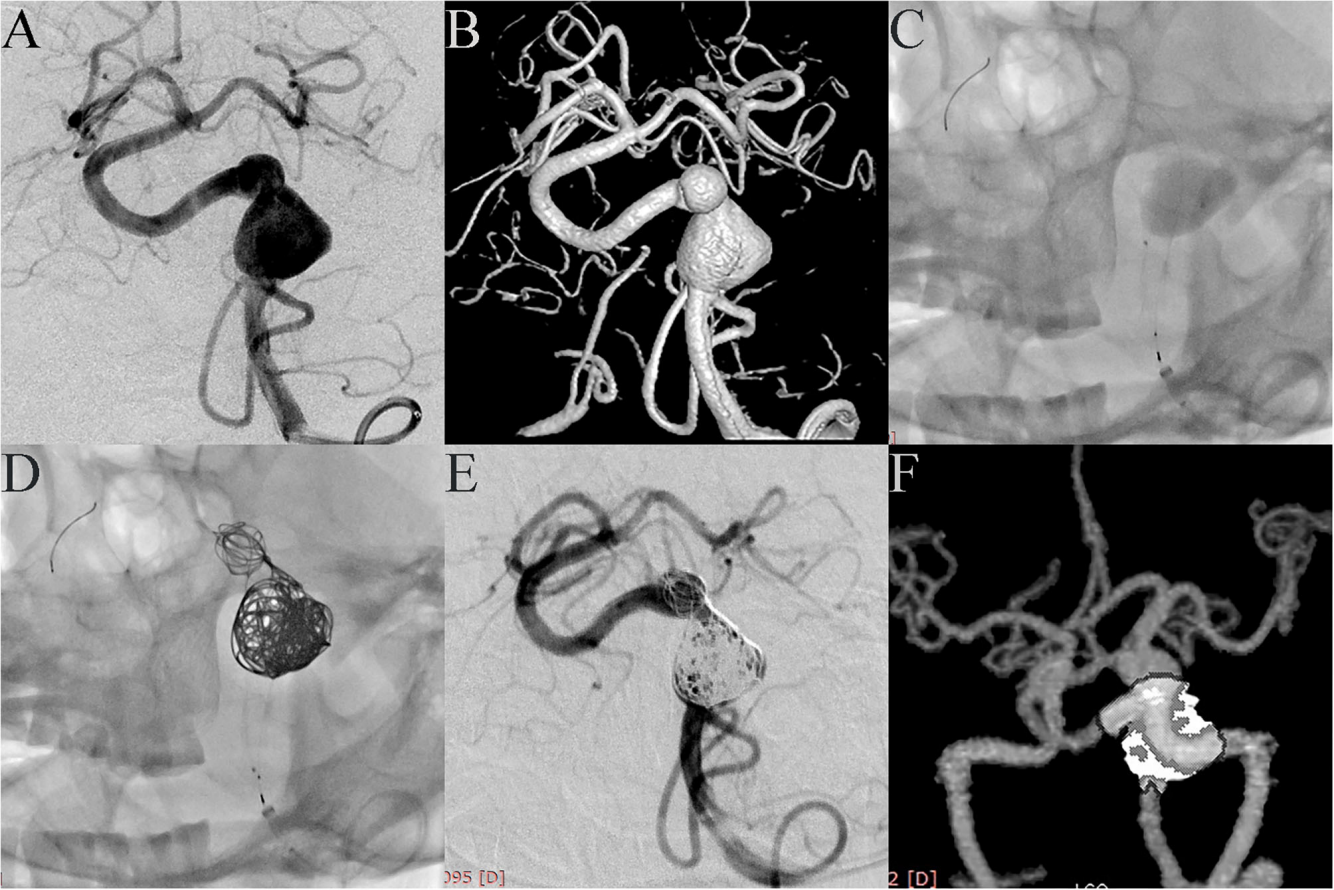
Case 4: Two adjacent fusiform aneurysms were found in the left vertebral artery. (A, anteroposterior projection by DSA; B, three-dimensional reconstruction). One PED was deployed along the left vertebral artery (C), and both aneurysmal necks were completely covered. Two aneurysm domes were then packed with coils (D). Post-embolization angiograms showed good vascular reconstruction. The large aneurysm was almost completely embolized and the small aneurysm showed significant contrast stasis (E). Follow-up at 10 months with CTA showed complete reconstruction of the vessel with the aneurysm completely occluded (F).
